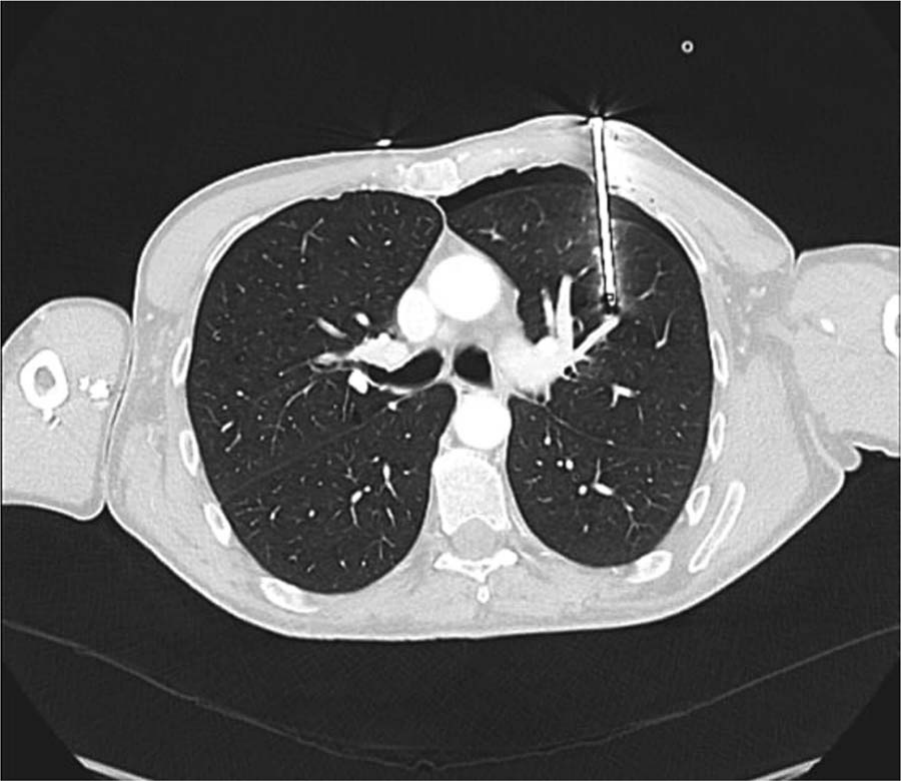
Axial CT chest demonstrating nail lodged in the left upper lobe, 3 mm from the anterior segmental branch of the pulmonary artery.
